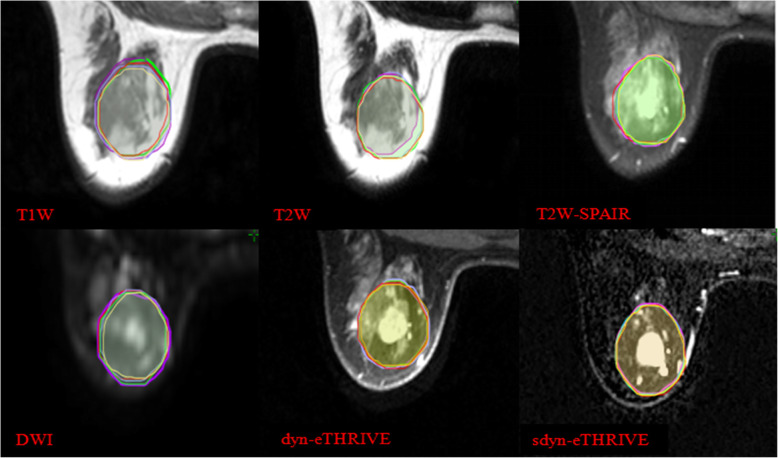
Delineations of CTV defined by 3 radiation oncologists and 2 radiologists in 6 pre-MRI sequences
